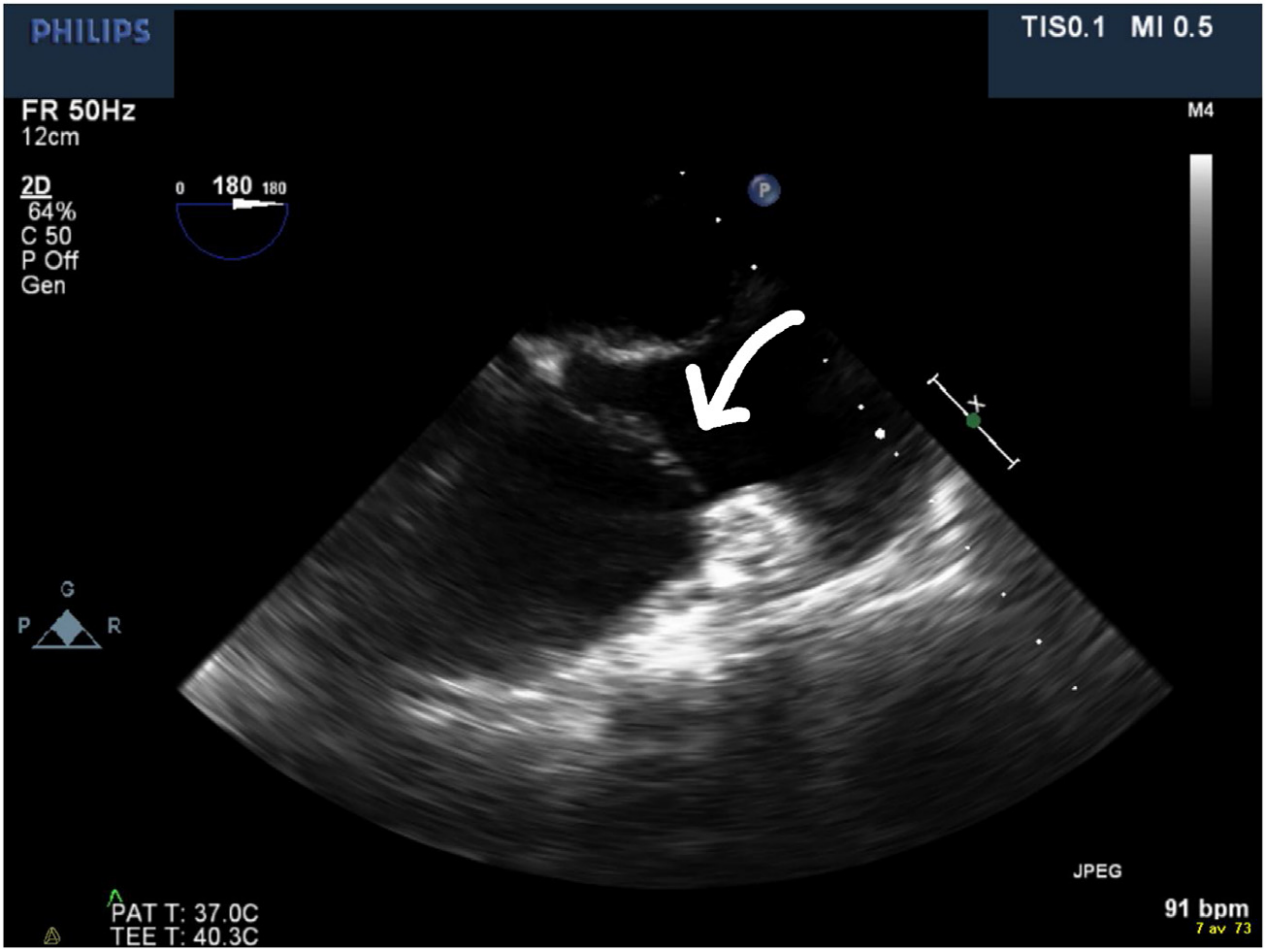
Transesophageal echocardiography five days after Fig. 1. No vegetation can be seen.
